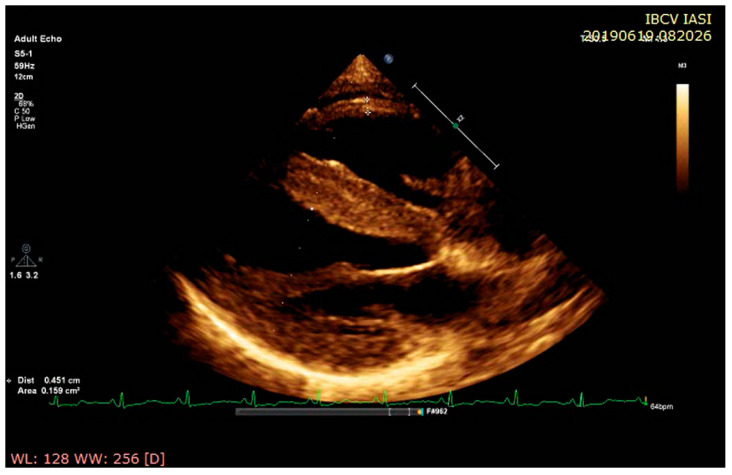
Echocardiographic assessment at rest in a young female with ATTR amyloidosis: parasternal long-axis view demonstrating concentric left ventricular pseudohypertrophy with concomitant right ventricular free wall hypertrophy.
